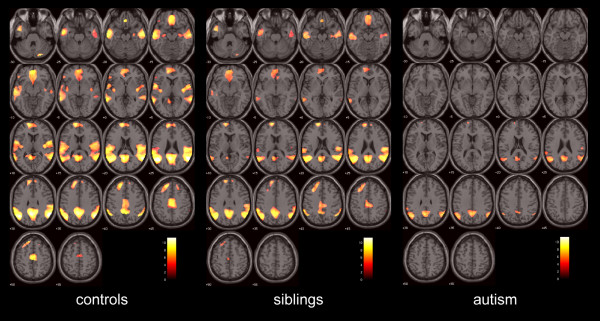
Task-related deactivations - the neural response to the control task versus the Embedded Figures Task condition, in controls (n = 40), unaffected siblings (n = 40) and adolescents with autism (n = 38). Activation maps indicate neural response to CT versus EFT conditions, corrected for multiple comparisons at whole brain *P* <0.05 FWE-corrected overlaid onto the canonical MNI152 template brain image (axial section, *z*-coordinate indicated in MNI space), with the colored bar indicating the T-value of the plotted activation differences. CT, control task; EFT, Embedded Figures Task; FWE, family-wise error; MNI, Montreal Neurological Institute.
